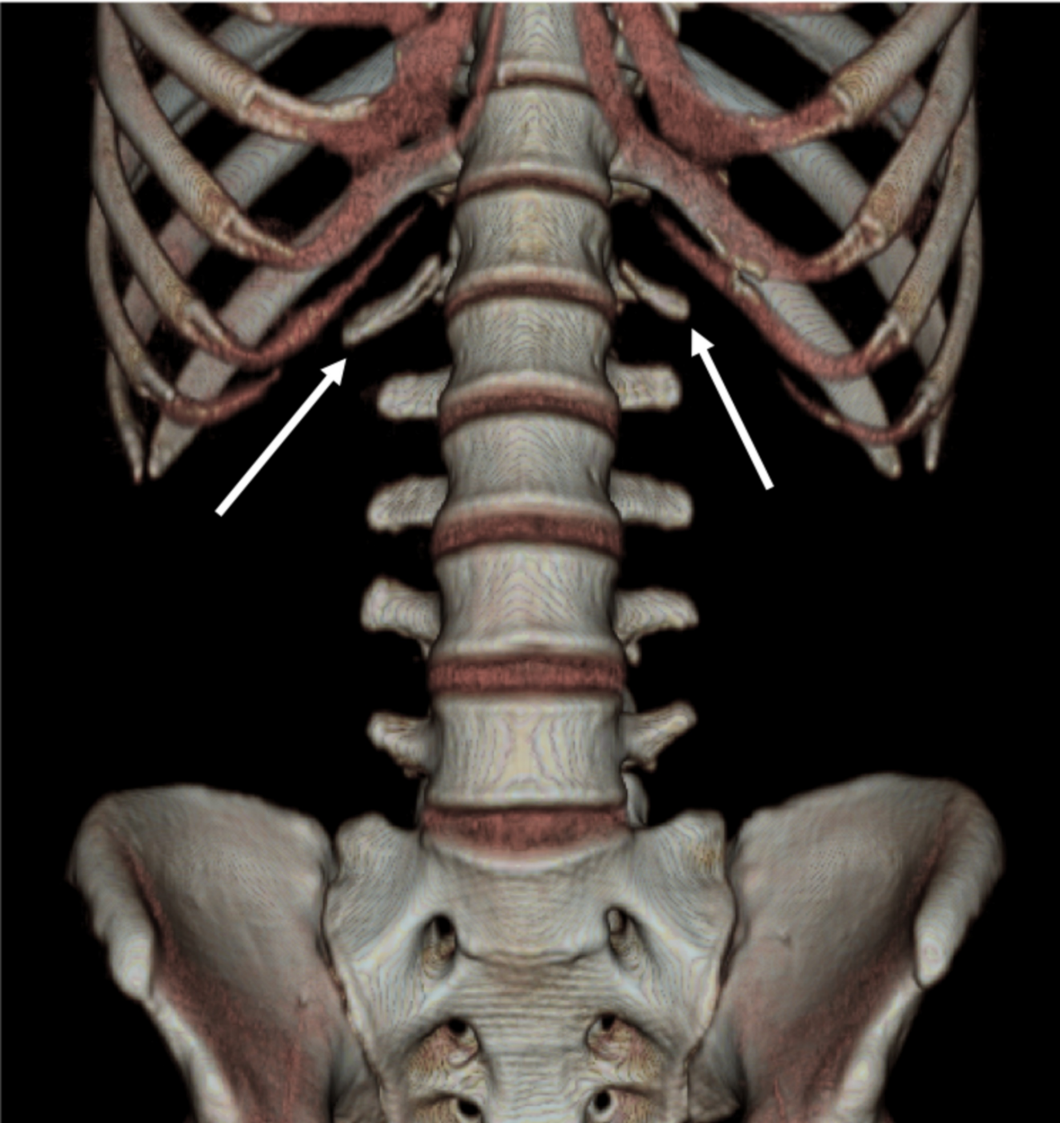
Lumbar ribs 3D computed tomography showing lumbar ribs (arrows) at L1. They are noticeably shorter and more horizontal compared to the thoracic ribs [15]
